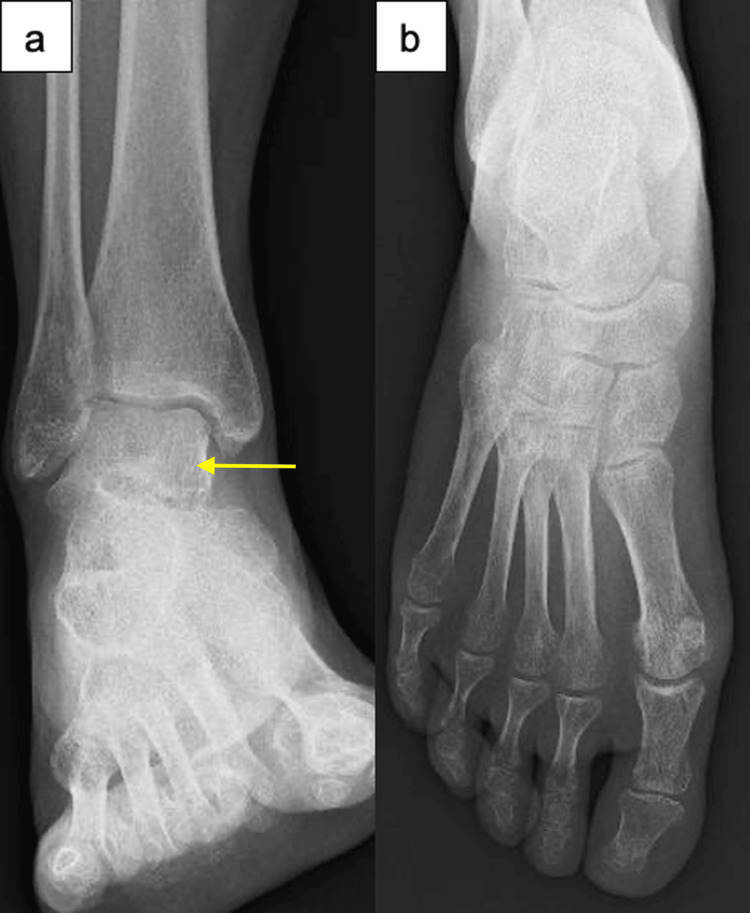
Serial anteroposterior radiographs of the right ankle and foot at 3 months postpartum. At three months postpartum: (a) partial resolution of osteopenia in the ankle; (b) improved bone density in the tarsal and metatarsal bones. Arrow indicates a representative area of improved bone density in the talus.
